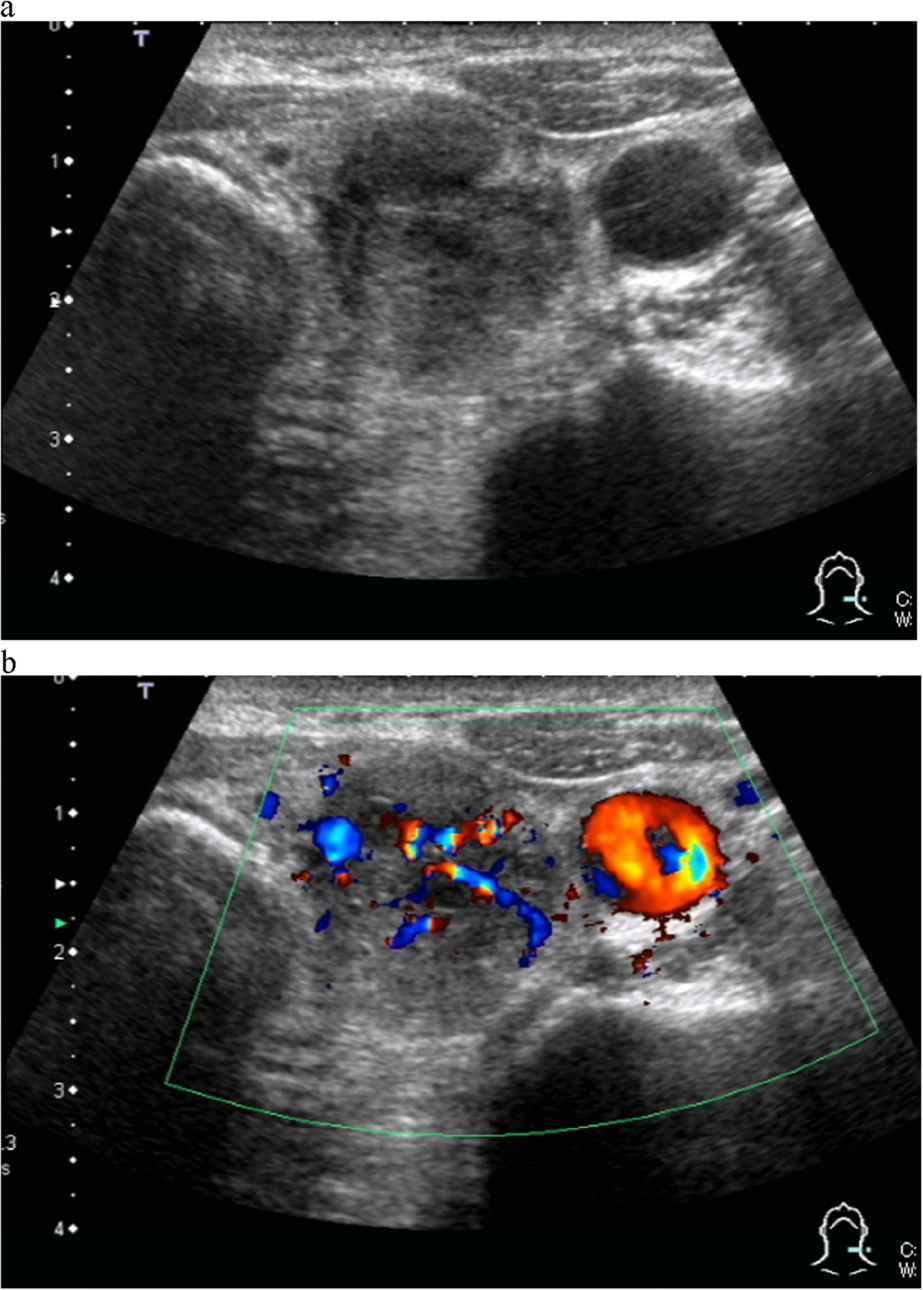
Neck ultrasonography. a Hypoechoic mass measuring 21 mm in size with irregular and partly indistinct margins, D/W ratio > 1. b Admission of abundant blood flow
